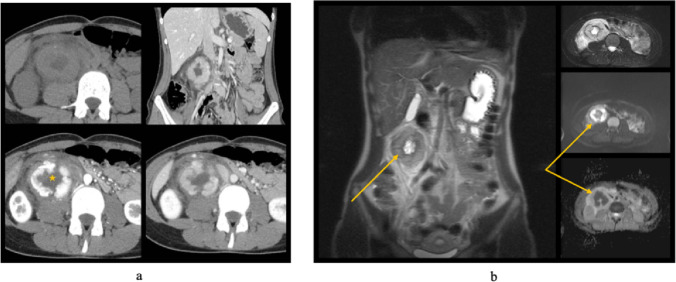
Retroperitoneal Inflammatory Myofibroblastic Tumor. Axial non-contrast, axial contrast-enhanced arterial and portal venous phase and coronal contrast-enhanced CT images (a) show a hypervascular mass with a hypodense necrotic center (orange asterisk) displacing the right kidney. MRI including coronal T2-weighted, axial fat-saturated T2-weighted, diffusion weighted (b100) and apparent diffusion coefficient images (b) show high restriction diffusion in the solid components of the mass (orange arrows). Reproduced with permission: [34]
